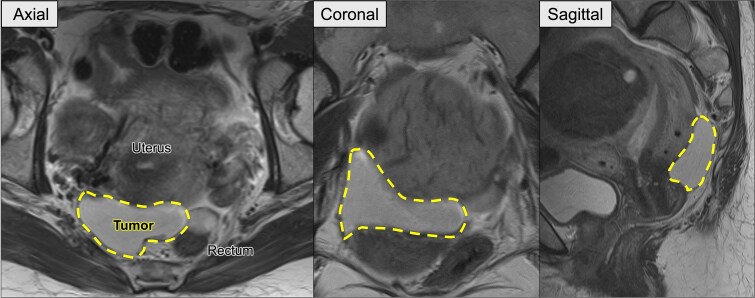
Preoperative pelvic MRI showing the recurrent retroperitoneal tumor (dashed line). Axial view: The tumor is located posterior to the uterus and anterior to the rectum. Coronal view: The tumor extends horizontally across the pelvic cavity. Sagittal view: The tumor is situated between the bladder and rectum, occupying the posterior pelvic space.
